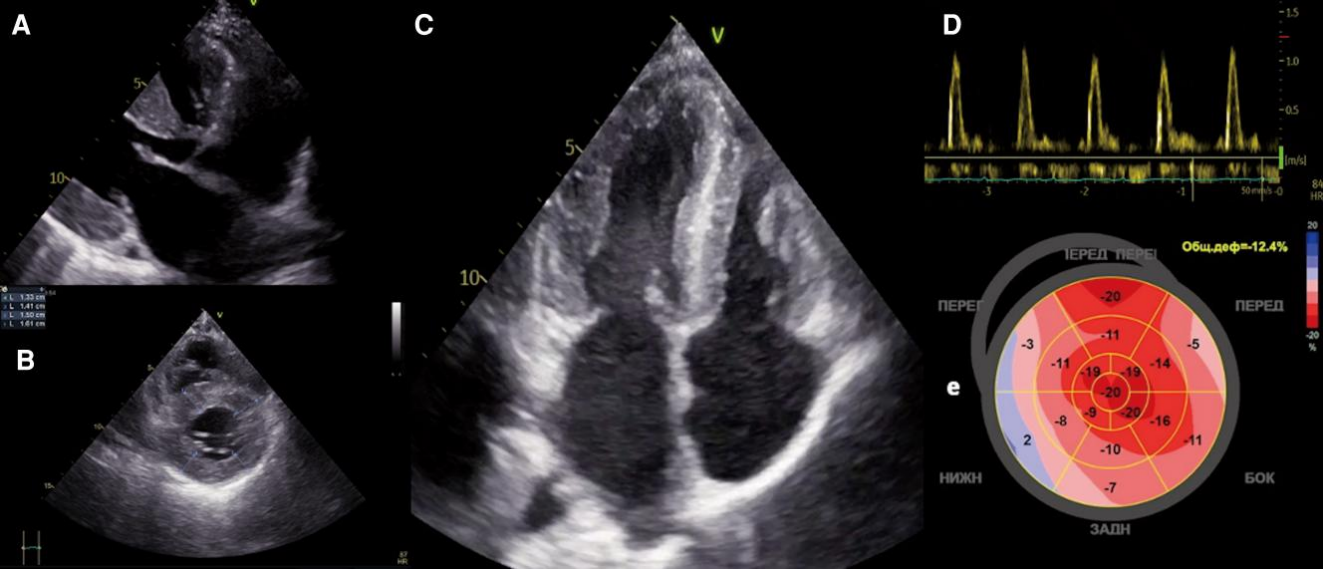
Echocardiogram shows (*A–B*) concentric LV hypertrophy with a maximal wall thickness of 15 mm, (*C*) reduced LV end-diastolic volume (25 mL/m^2^) and mild left atrial enlargement (35 mL/m^2^), (*D*) markedly elevated E/A ratio on mitral inflow Doppler (4.7), and (*E*) decreased global longitudinal strain (12.4%) with a ‘cherry on top’ pattern.
